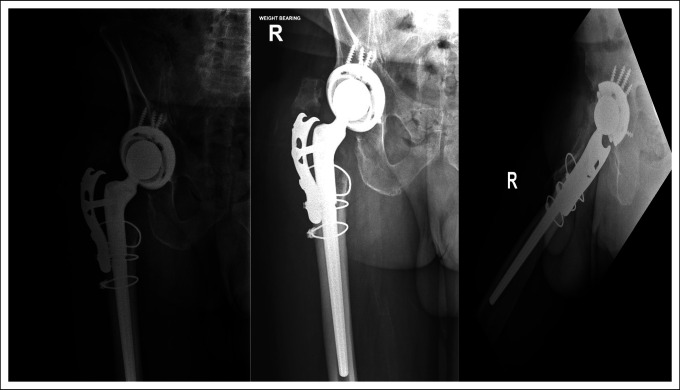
Radiographs from the 1-month follow-up on June 04, 2021.
